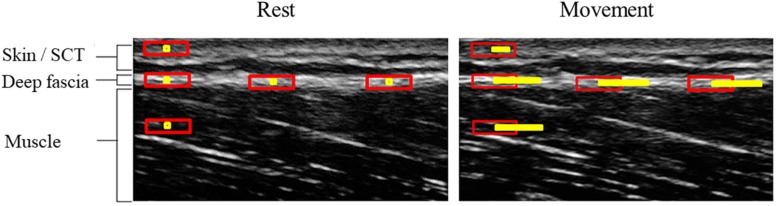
Exemplary visualization of ultrasound-based cross-correlation analysis. Equidistant ROIs (red rectangles) are selected at rest (*left image*). In this example, five ROIs have been positioned: three within the fascia and one in each, the subcutaneous tissue (SCT) and the muscle. Upon movement (*right image*), pixel displacements relative to the center of the non-moving ROIs (small yellow square inside the red rectangles in left picture) are tracked (yellow line in right picture). The end of the line indicates the maximal displacement, which is computed by the software algorithm.
